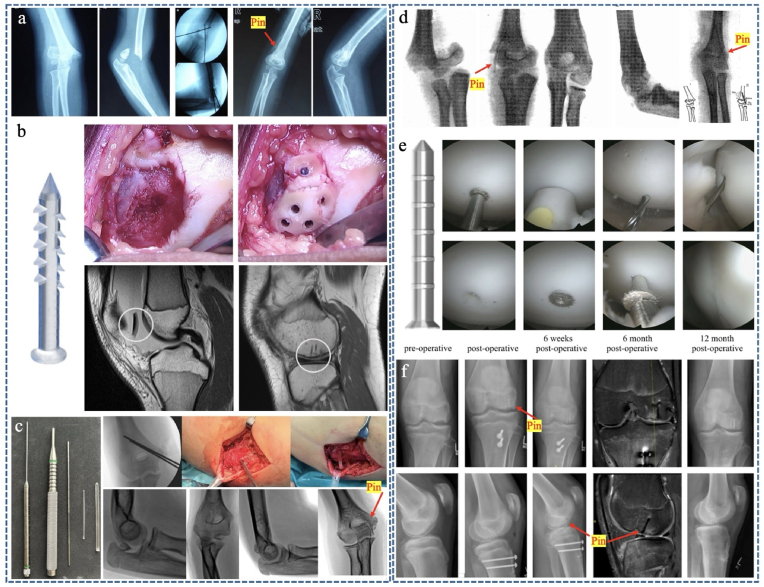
(a) X-ray showed Gartland type III supracondylar fracture of the humerus in a 5-year-old girl, once reduction of the fracture and location of the pins were confirmed satisfactory, the K-wires were replaced with the GRANDFIX™ PDLLA bioabsorbable pins through the primary pin tracks. Reproduced with permission from Ref. [164]. Copyright 2011, Springer. (b) Intraoperative images of two pieces of the osteochondral fracture (OCF) fixated with six bioabsorbable PLA SmartNails, Preoperative MRI of the OCF fragment and postoperative MRI showing OCF healed. Reproduced with permission from Ref. [171]. Copyright 2022, SAGE. (c) Medial epicondyle avulsion fractures in an 11-year-old girl were reduced and stabilizated with K-wires: under fluoroscopy; If the wires were in an optimal position, then they were replaced with PLGA biodegradable pins. Reproduced with permission from Ref. [168]. Copyright 2022, Wolters Kluwer Health. (d) Lambotte and Verbrugge shows a supracondylar humerus fracture of a child fixated using a magnesium pin, and the early onset of subcutaneous gas formation can be seen. Reproduced with permission from Ref. [104]. Copyright 2010, Elsevier. (e) Arthroscopic insertion of the pin and (f) Fixation of a displaced osteochondral fragment of the lateral femur condyle after patella dislocation with 3 pins and realignment of the patella using the Elmslie-Trillat procedure in a 16-year-old boy. Reproduced with permission from Ref. [174]. Copyright 2021, SAGE.
